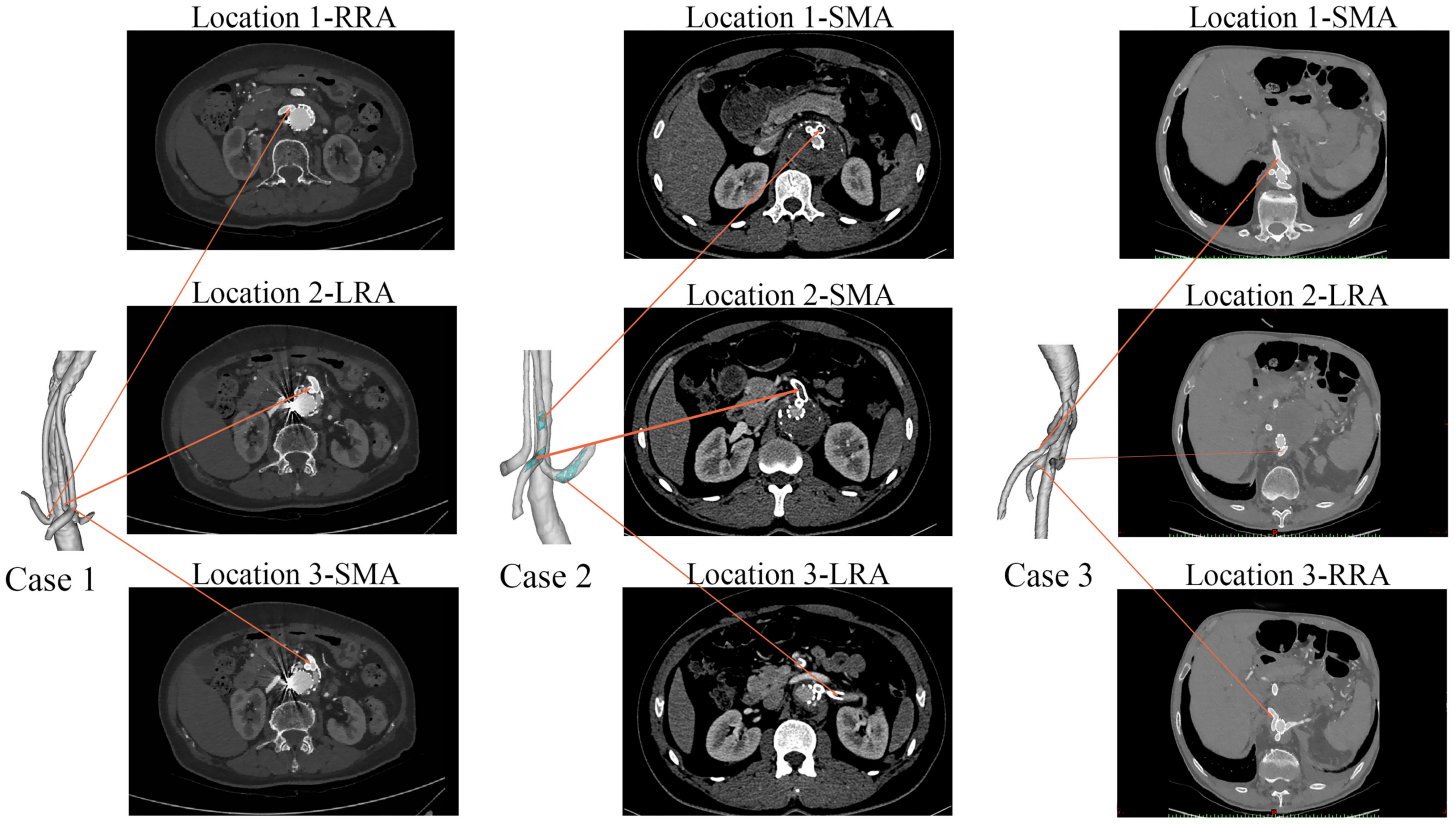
The follow-up CT scans of the three cases. Case 1 had no thrombi in the branches, Case 2 had branched stent-graft thrombosis (marked in green), and Case 3 had no thrombi in the branches.
